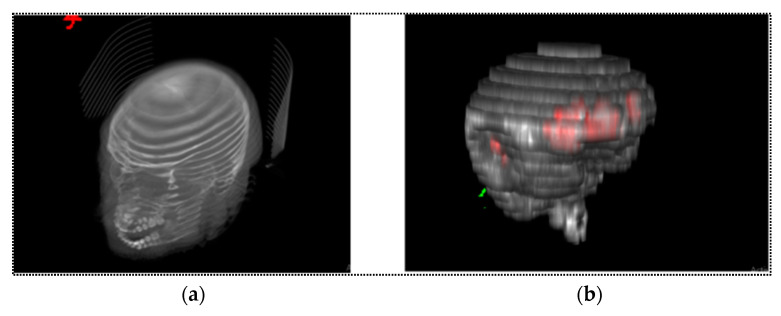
Volumetric rendering: (a) head slices including skull and brain and (b) after skull-stripping from the same patient’s CT images (The intracranial hemorrhage is highlighted with red color).
